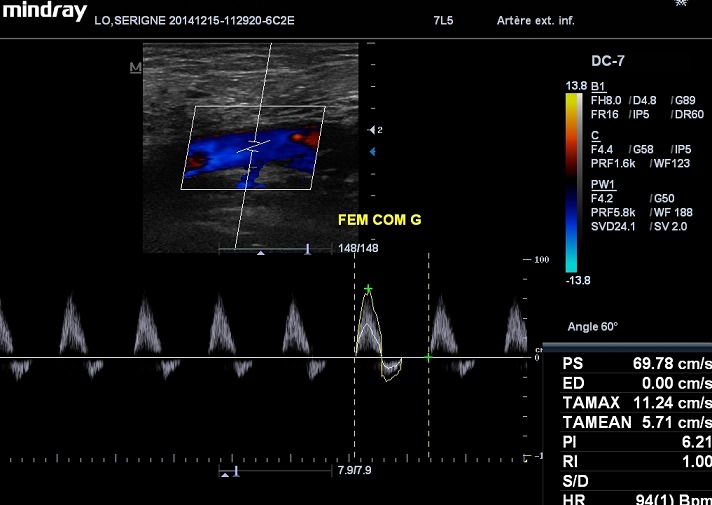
Spectre Doppler d'une sténose non serrée (‘75%) d'une artère fémorale commune avec une accélération modérée des vitesses systoliques et aliasing
